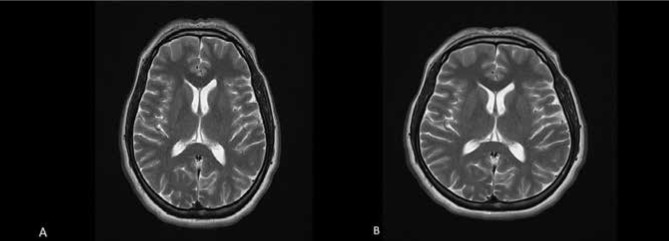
Comparison of brain MRI T2-weighted 2D TSE axial section images in the original protocol and after changing parameters. A) Original protocol: scan time (TA) of 3.47 minutes, FOV of 230 mm, 90% FOV phase, and 0% phase oversampling. B) Modified protocol: scan time (TA) of 2.18 minutes, FOV of 217 mm, and 93.98% phase oversampling of 13.96%.
